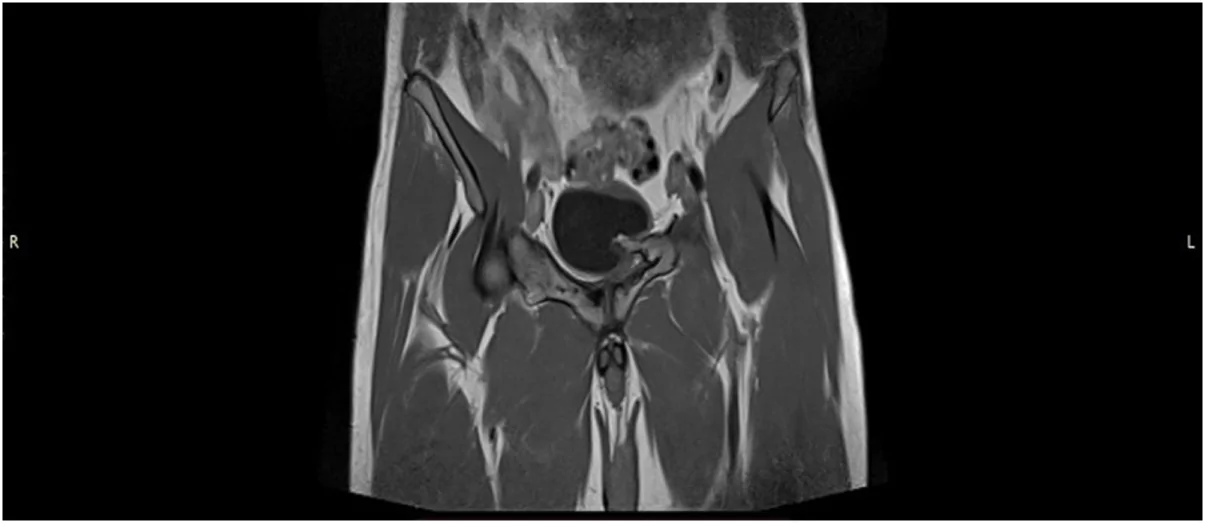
Pelvic MRI (T2-weighted coronal sequence) demonstrating a 1.7-cm exophytic bony protuberance arising from the upper cortex of the left pubic ramus, abutting and compressing the left lateral bladder wall, with adjacent reactive wall thickening, consistent with an inflammatory response to chronic mechanical compression.
